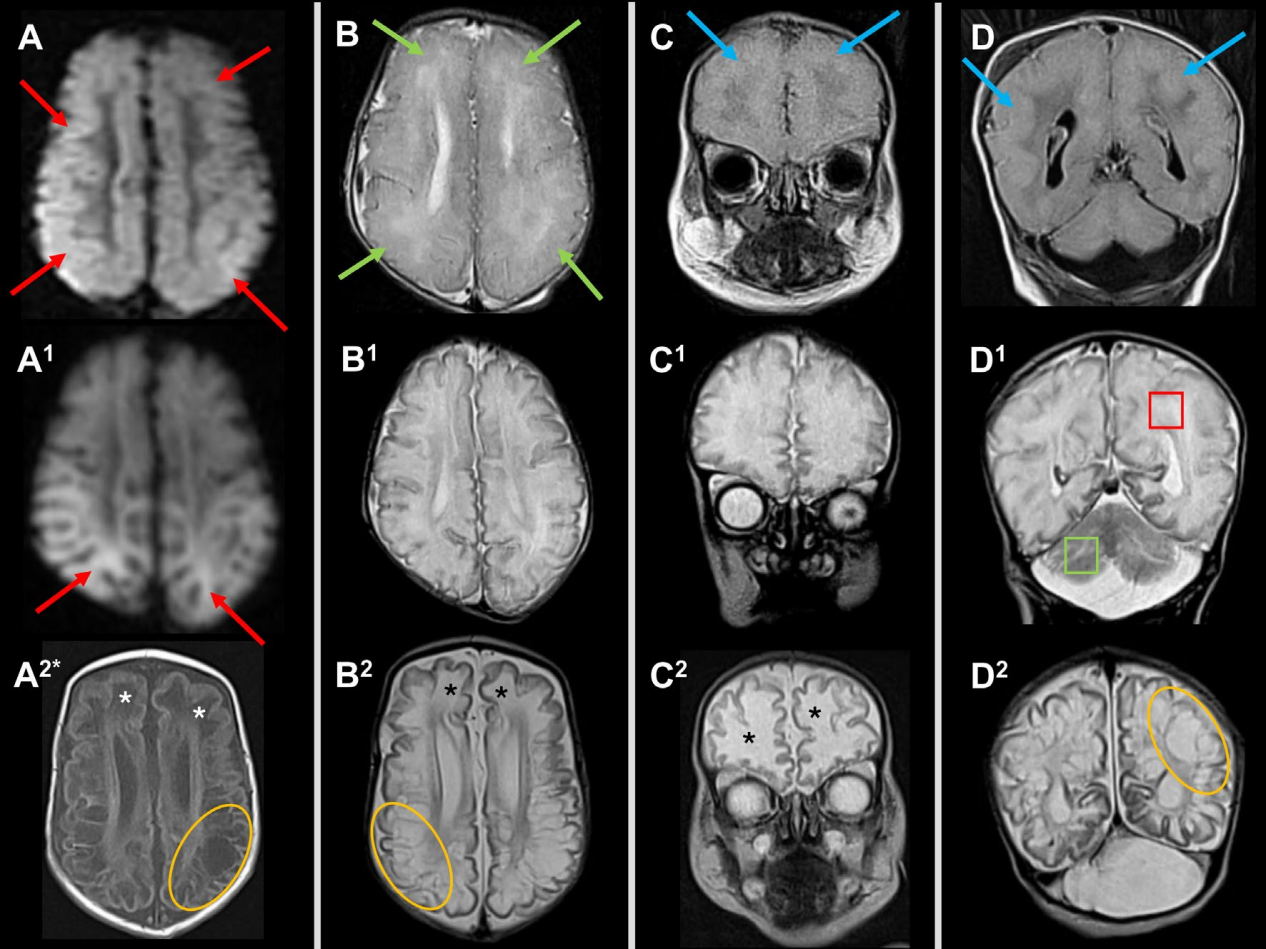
Transition from cytotoxic edema through liquefaction to cystic transformation in an infant with MoCD (Patient D). Upper row (A–D): At age 6 days; Middle row (A1–D1): At age 10 days; Bottom row (A2*–D2): At age 21 days. 1st column: (A–A1): Axial DWI, (A2*): Axial T1WI. DWI sequence was not obtained at age 21 days. 2nd column: (B–B2): Axial T2WI of the frontoparietal lobes. 3rd column: (C–C2): (C) Coronal T2 FLAIR; (C1–C2) Coronal T2WI through the frontal lobes. 4th column: (D–D2): (D) Coronal T2 FLAIR; (D1–D2) Coronal T2WI through the parietal, posterior temporal, and occipital lobes. A–A1: High DWI signal/restricted diffusion in the frontoparietal cortex and subcortical white matter reflect cytotoxic edema at age 6 and 10 days (red arrows). Note restricted diffusion persists with posterior gradient, more striking in the posterior frontal and parietal lobes at age 10 days (A1). B–D: Mildly reduced cortico‐subcortical T2 signal (green arrows)/increased T2 FLAIR signal (blue arrows) compared to deep WM in the swollen cerebral hemispheres with loss of cortical ribbon and sulcal effacement at age 6 days. Note increased water content in deep WM reflecting vasogenic edema. B1–D1: Reduced degree of brain swelling with visualization of previously effaced cerebral sulci. Note high T2 signal in the swollen cerebral white matter (red quadrant) compared to lower T2 signal intensity in the cerebellum (green quadrant); (D1). B2–D2: High uniform T2 signal in the anterior frontal lobes compatible with CSF signal along the sulci represents liquefaction (black asterisks)—a pre‐stage before cystic transformation (B2, C2). Simultaneously, cystic transformation occurred earlier in the posterior frontal, parietal, temporal, and occipital lobes (orange ovals) (B2, D2). Note interval cerebral atrophy with prominent CSF spaces along the sulci and dilated lateral ventricles at age 21 days. A2*: Low T1 signal in the anterior frontal lobes reflects liquefaction (white asterisks), while cystic transformation occurred with posterior gradient in the parietal and posterior frontal lobes (orange oval).
